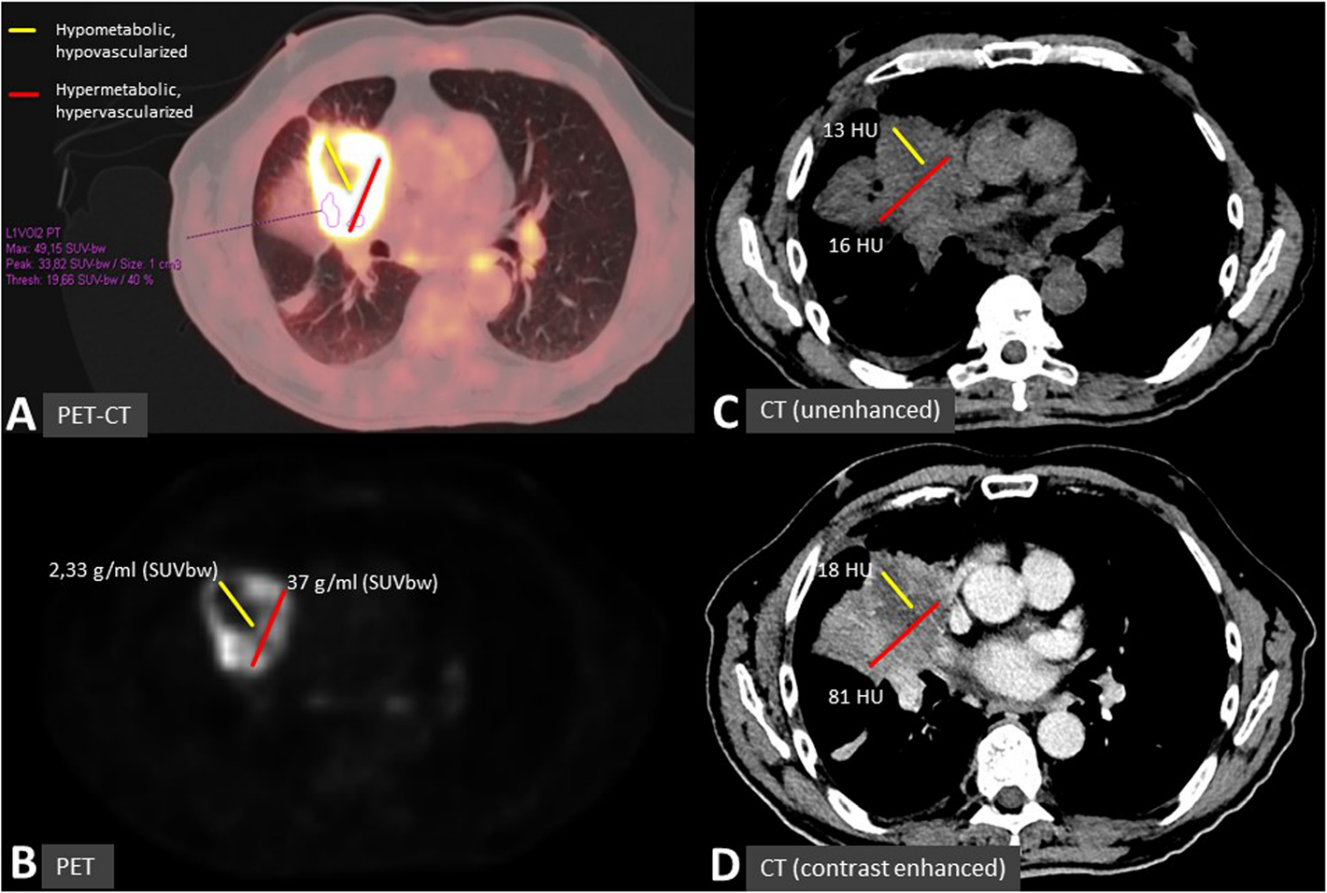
a-d Definition of target for SBRT-PATHY: BTV, or “hypoxic“ tumor segment refers to the combined 18F-FDG PET-CT (a, b) and contrast-enhanced CT (d) image findings, derived from the SUV and HU values. This to capture the junctional, contrast-hypo-enhanced (i.e. no significant increase in HU-values after contrast medium injection: c vs. d) and hypometabolic (SUV-value of ≤3 and/or ≤ 30% of SUVmax) tumor regions between the central-necrotic and the remaining peripheral-vascularized and hypermetabolic tumor segments
